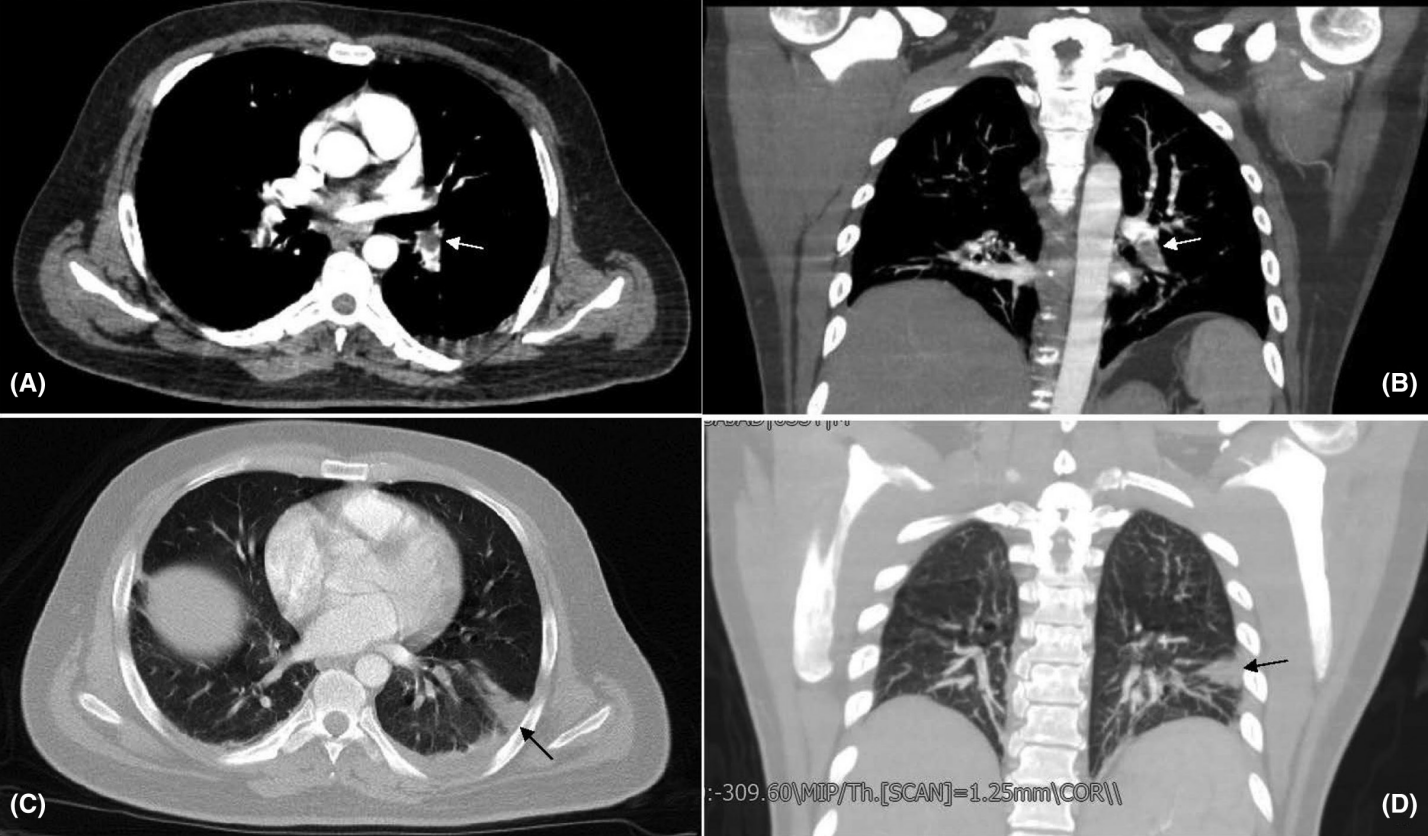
CT pulmonary angiogram (CTPA) revealed a filling defect at the bifurcation of the left pulmonary artery extending to the left lower lobe artery, consistent with arterial embolism (A and B, white arrows). A focus of wedge‐shaped infarction is evident at the lateral basal segment of the left lower lobe (C and D, black arrows).
